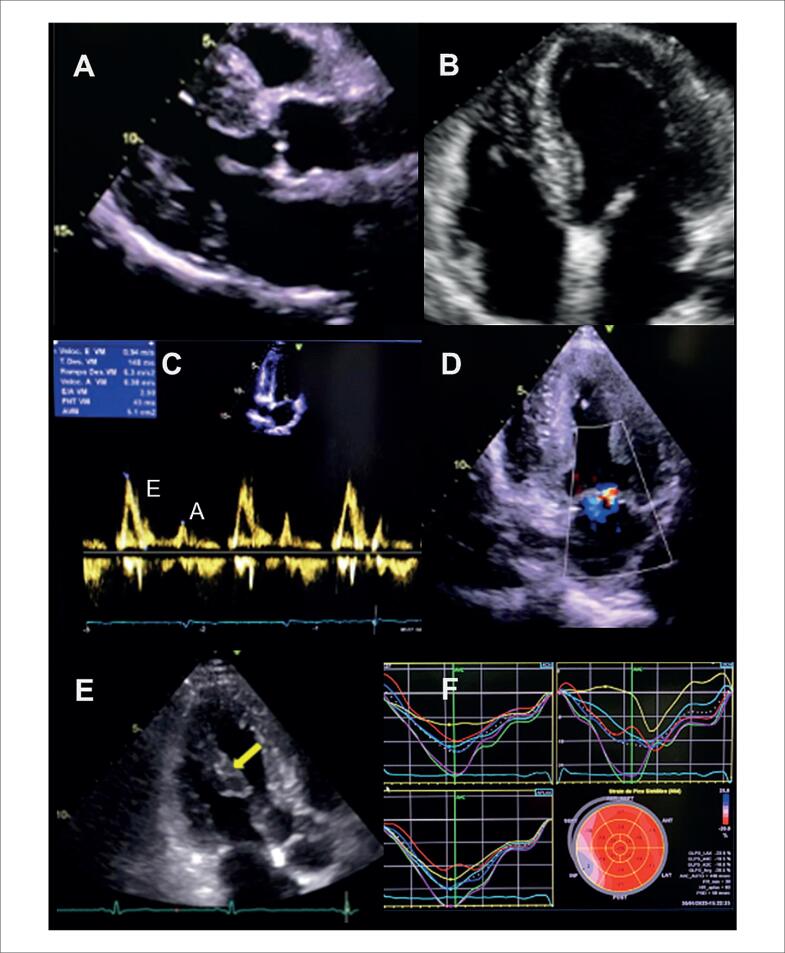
Achados ecocardiográficos na doença de Fabry (DF). A) Janela paraesternal longitudinal evidenciando aumento da espessura das paredes do ventrículo esquerdo. B) Janela apical quatro câmaras evidenciando o sinal binário endocárdico (aspecto de birrefringência do miocárdio) em parede septal inferior. C) Doppler do fluxo transmitral com padrão de disfunção diastólica importante (relação E/A > 2). D) Janela apical quatro câmaras evidenciando refluxo mitral discreto. E) Janela apical três câmaras evidenciando aumento da espessura de músculo papilar (seta amarela). F) Representação das curvas de strain longitudinal segmentar e mapa paramétrico com alteração característica da DF evidenciando redução do strain segmentar em segmento basal da parede inferior.
